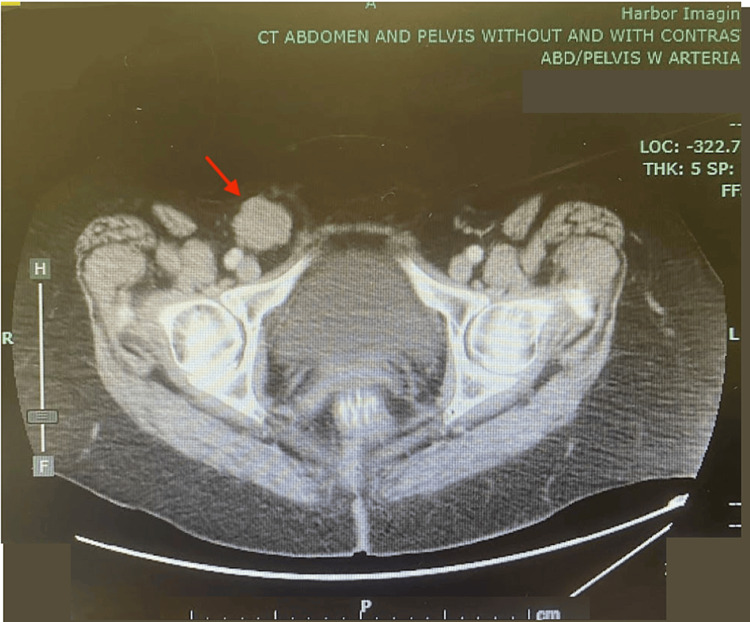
Preoperative CT of pathologic right inguinal lymph node
